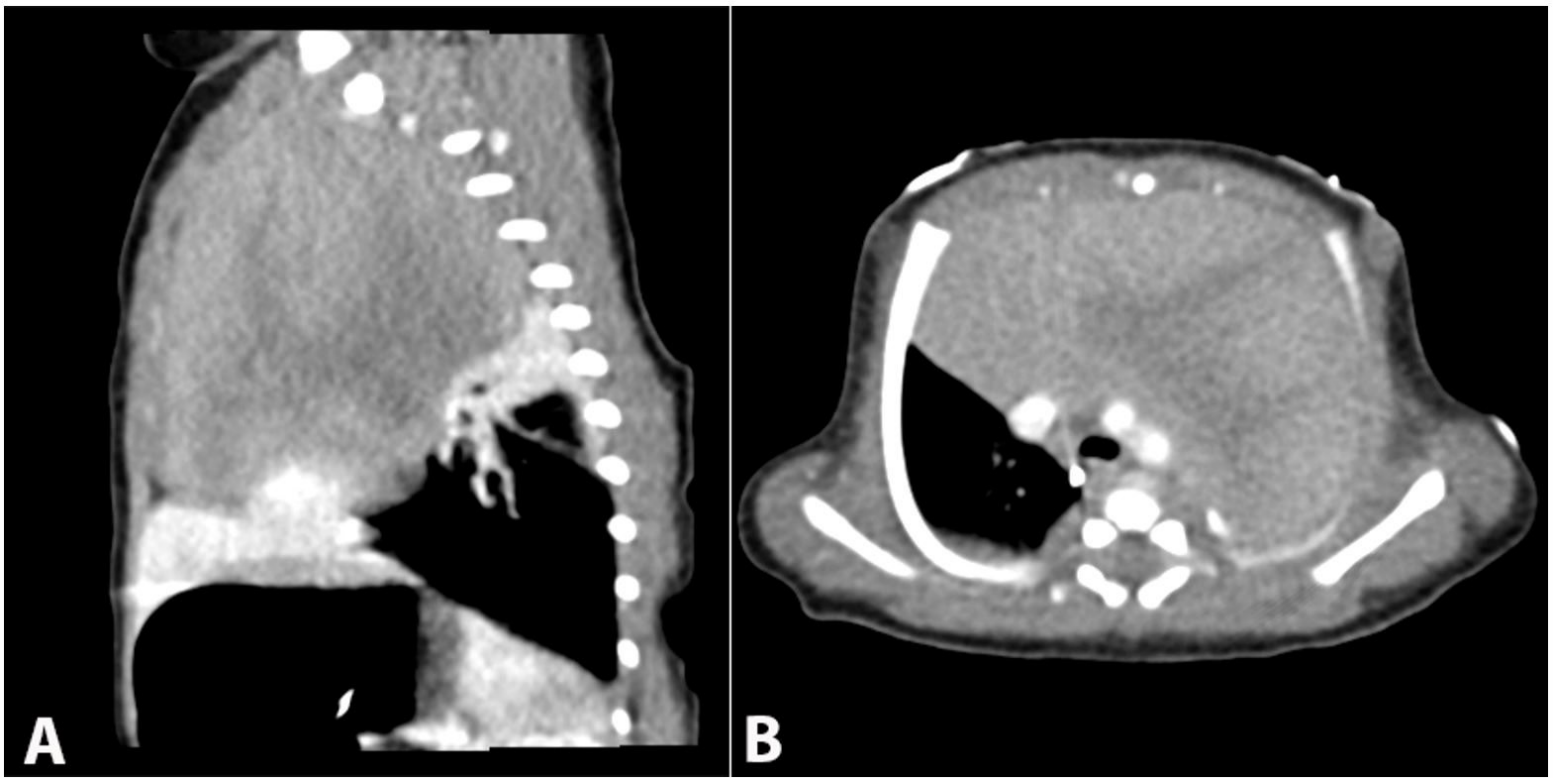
A – Sagittal contrast-enhanced thoracic computed tomography (CT) demonstrates a large, heterogeneously enhancing, anterior mediastinal mass. No calcification or necrosis is noted. The mass causes passive atelectasis of the adjacent right middle and upper lung lobes; B – Axial contrast-enhanced thoracic CT demonstrates a large anterior mediastinal mass with heterogeneous enhancement. The lesion exerts mass effect on the great vessels, displacing them posteriorly. Extrinsic compression on the tracheal is also noted, but the tracheal remains patent. No area of necrosis, calcification, or lymphadenopathy noted.
